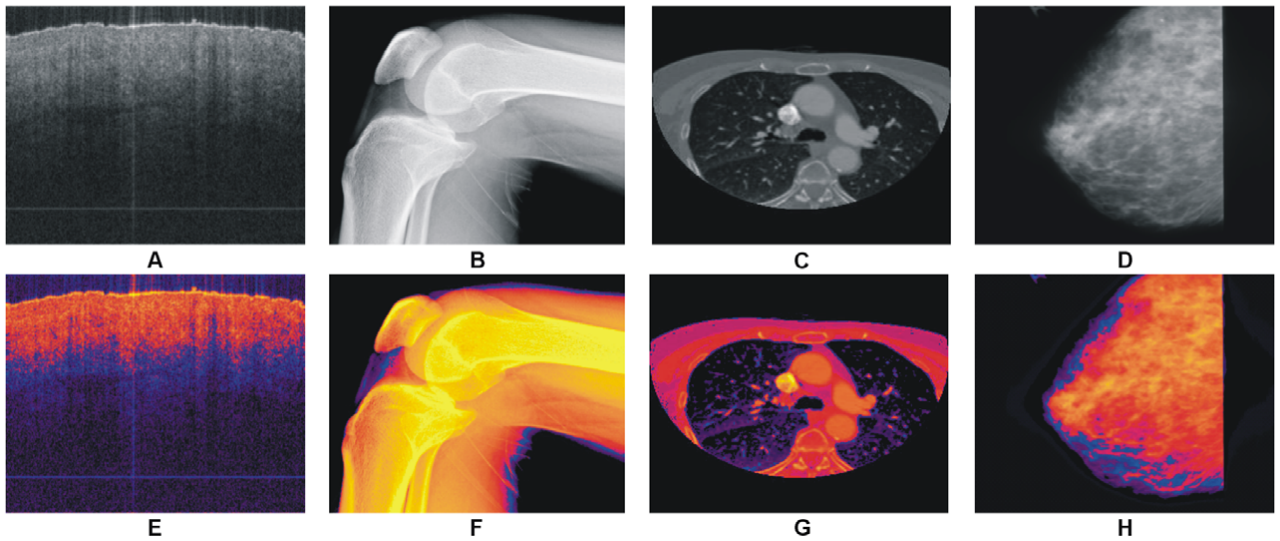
Results of our proposed colorization method with other medical imaging modalities. (A) OCT image of nail under skin. (B) Digital X-Ray of knee. (C) CT image of thorax. (D) Mammographic image. (E)–(H) Colorized images of (A)–(D) with our proposed method respectively.
